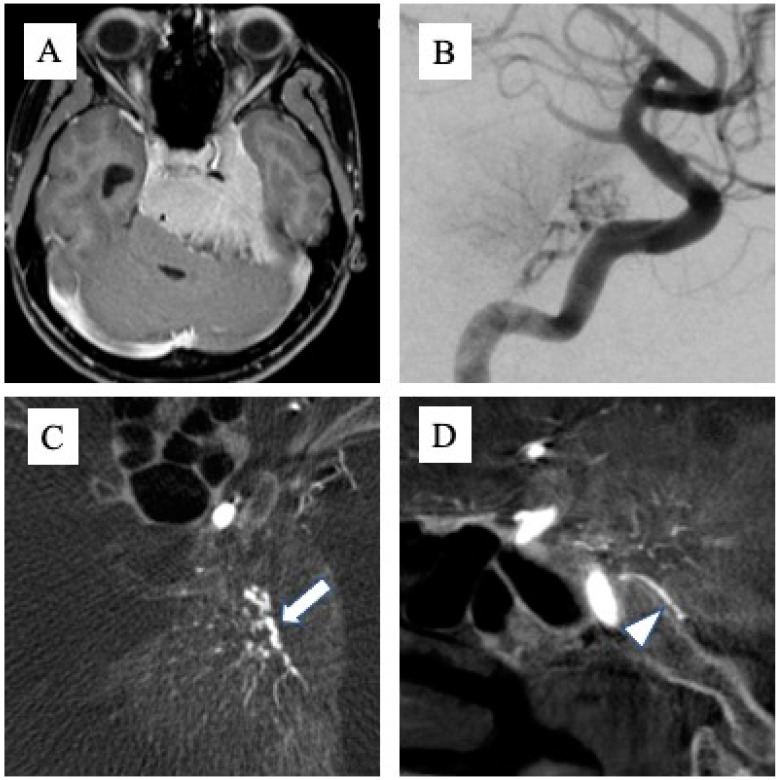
Cone-beam CT obtained from digital subtraction angiography. When the feeding artery was difficult to define via 2D angiography, cone-beam CT helped to determine the running course and accurately identify the vessel. (A) An example of a huge meningioma with broad attachment. (B) When various feeding arteries were visualized, it was difficult to define the accurate name of artery from 2D angiogram alone. (C,D) The anatomical relationships and running courses of the tentorial artery (C: arrow) and dorsal meningeal artery (D: arrowhead) were easy to understand from cone beam CT.
